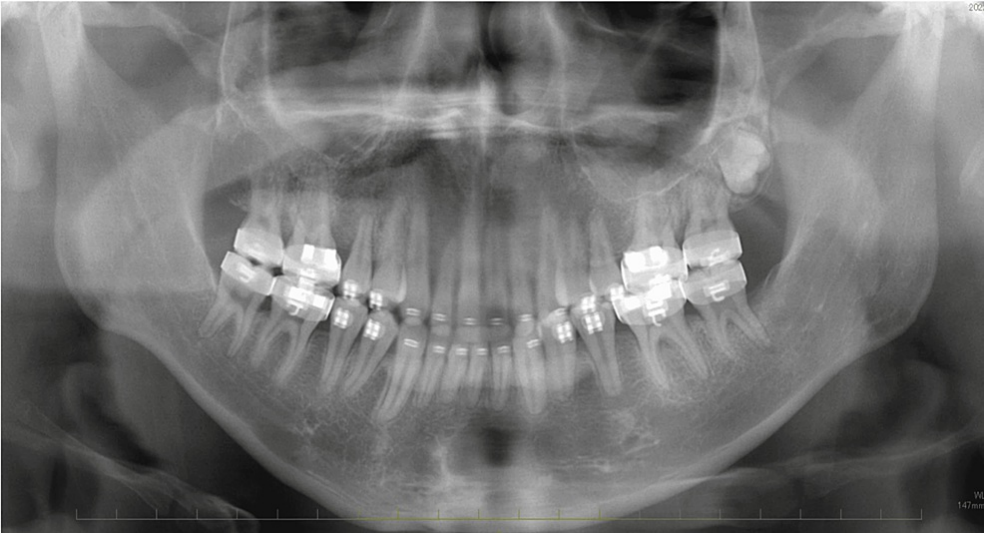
Presurgical evaluation. Panoramic radiograph.
